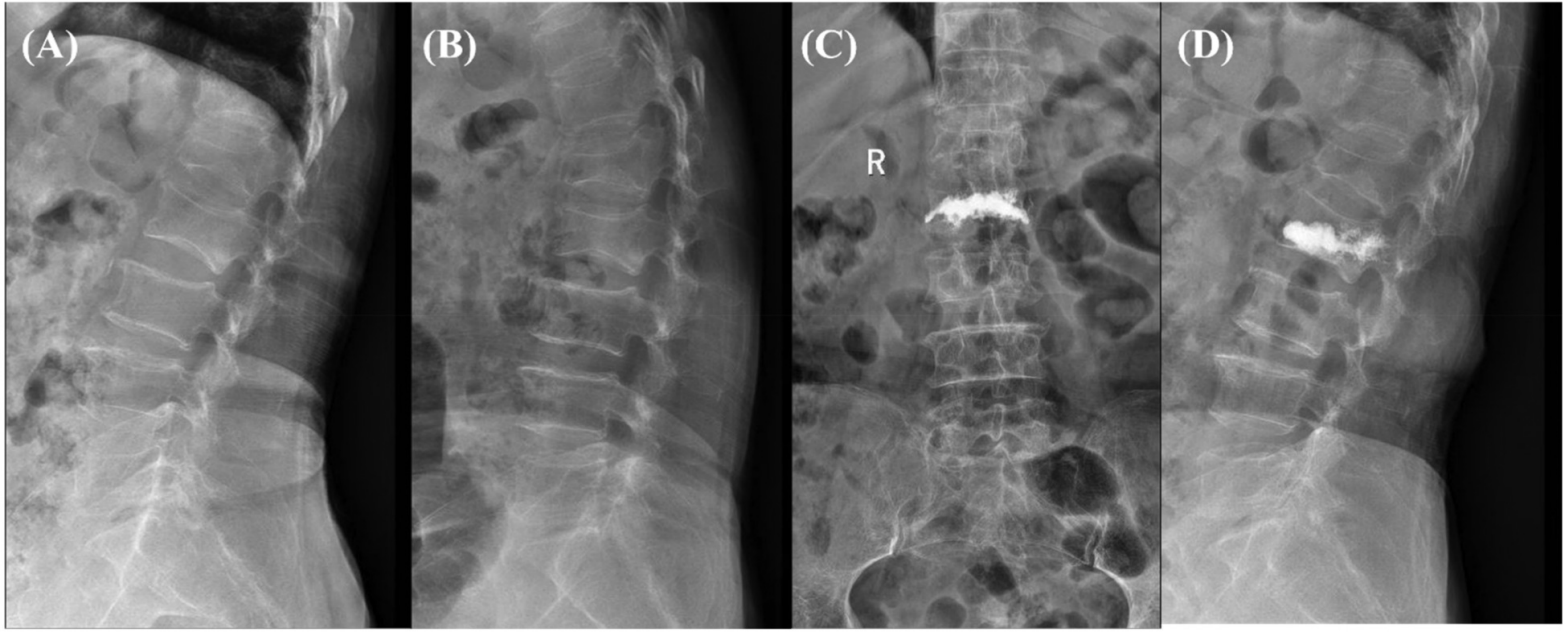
(A,B) preoperative x-ray showing an L2 vertebral compression fracture, characterized by significant vertebral body collapse and height loss. (C,D) Postoperative x-ray following vertebroplasty, demonstrating successful cement injection and filling of the fractured vertebra, with restored vertebral body height and complete cement filling. No complications, such as cement leakage, are observed. The patient showed good functional recovery post-surgery.
